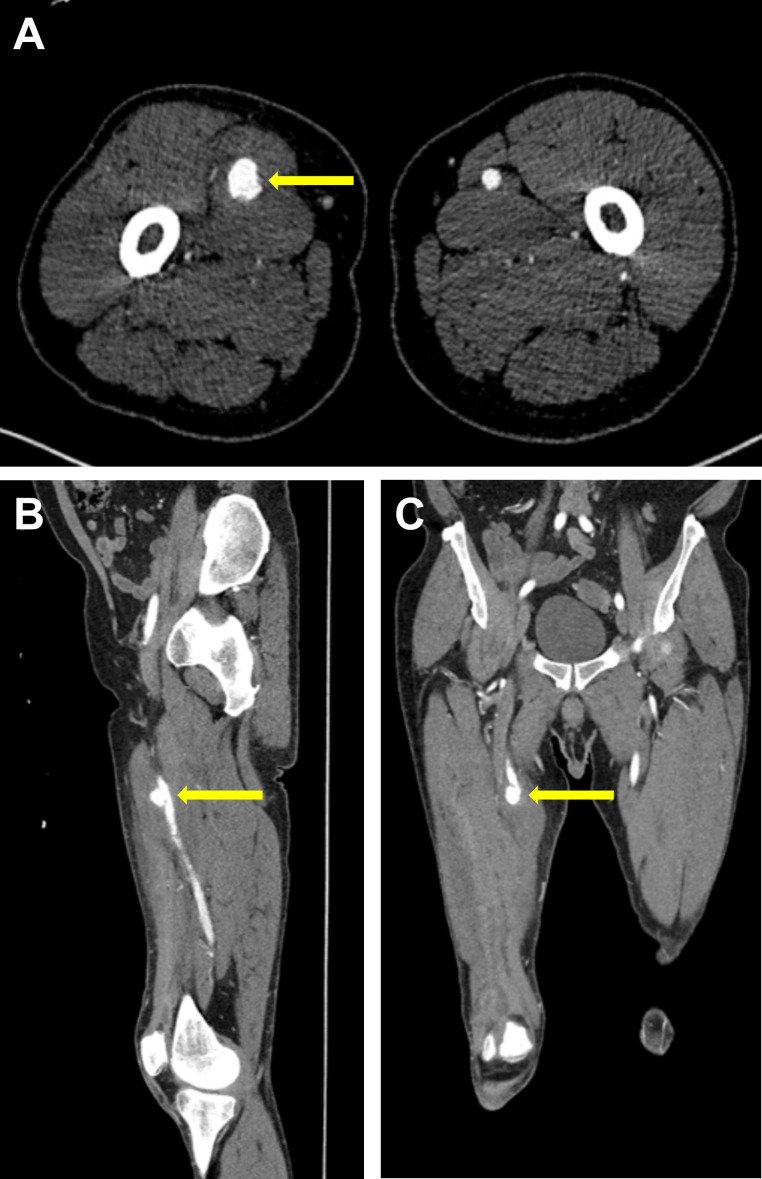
The patient’s initial computed tomographic scan showing the pseudoaneurysm of the right superficial femoral artery (yellow arrows) on representative axial (A), sagittal (B), and coronal (C) views.
